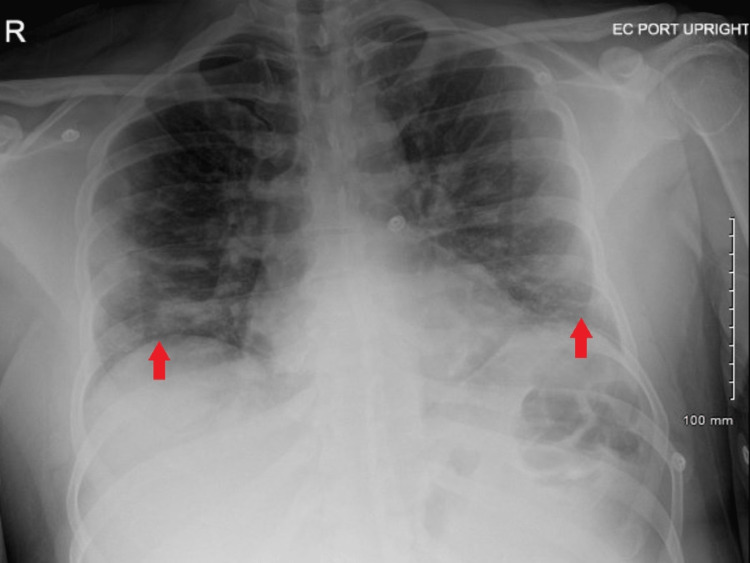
Chest X-ray Findings consistent with vague ill-defined opacities at the lung bases bilaterally, raising concern for atypical bilateral pneumonia (red arrows).
